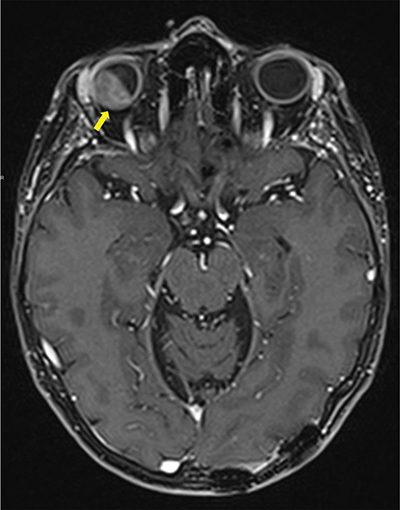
Contrast enhanced Axial T1 Dixon MRI-scan showing intra-orbital metastasis (arrow) invading all layers of the eyeball and the intra-conal fat.
